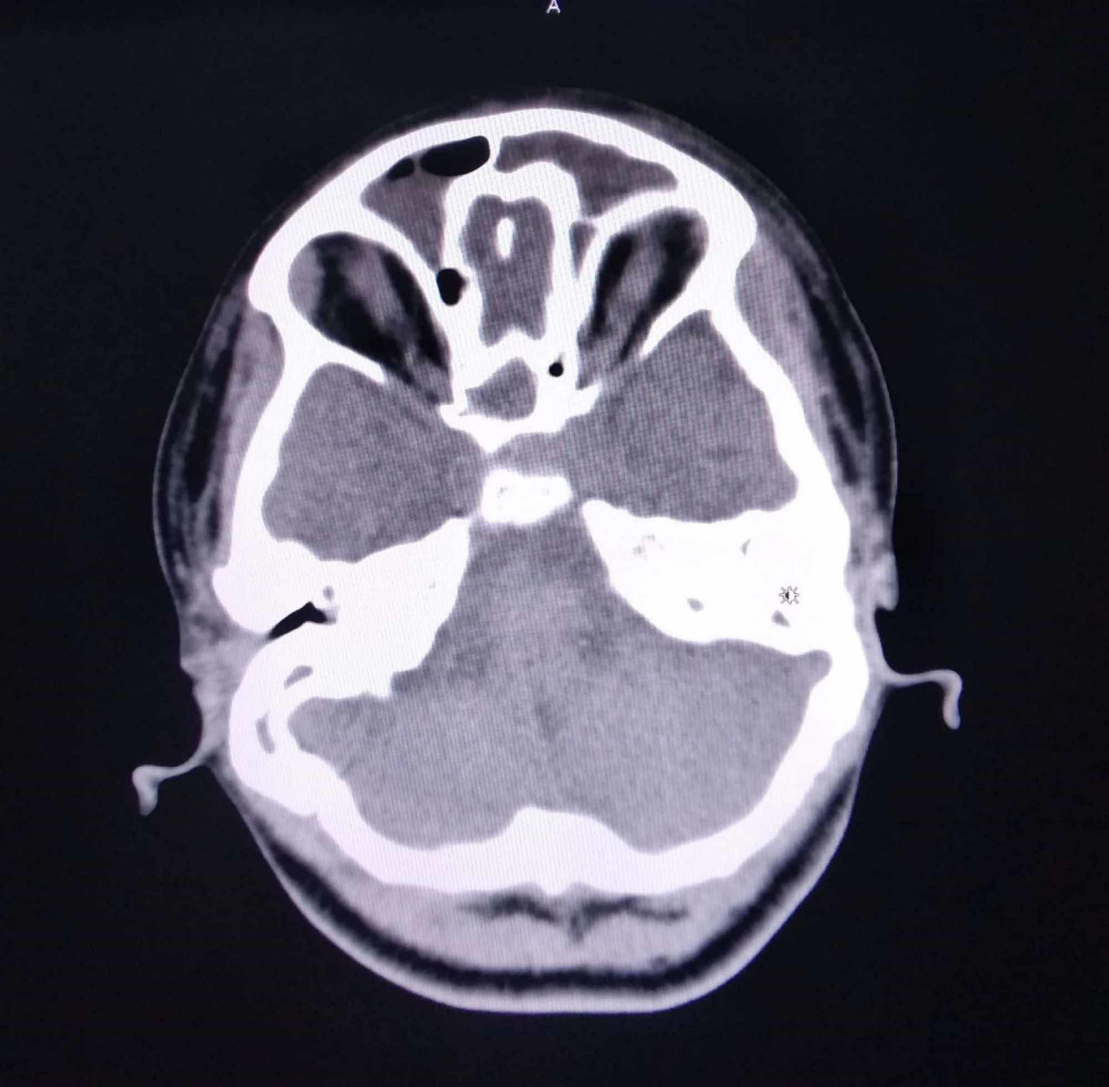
Axial CT scan, showing pansinusitis
